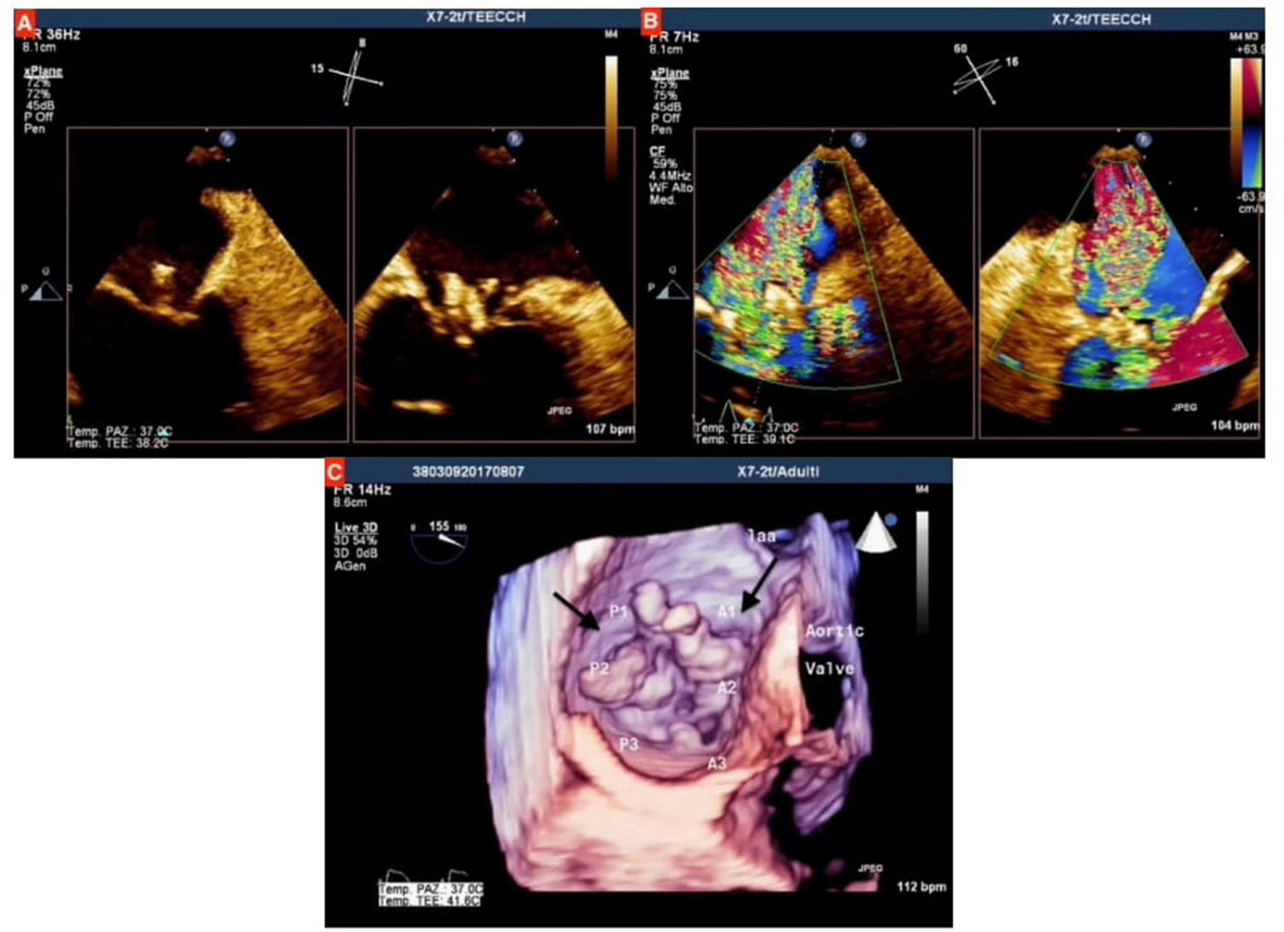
(A) Qualitative assessment of mitral valve at transesophageal echocardiogram (TEE), showing the two vegetations on both anterior and posterior mitral valve leaflets, which protrude toward left atrium during systole; (B) Assessment with color doppler showing severe mitral regurgitation; (C) three-dimensional assessment at TEE, the black arrows indicate the two vegetations on mitral leaflets.
